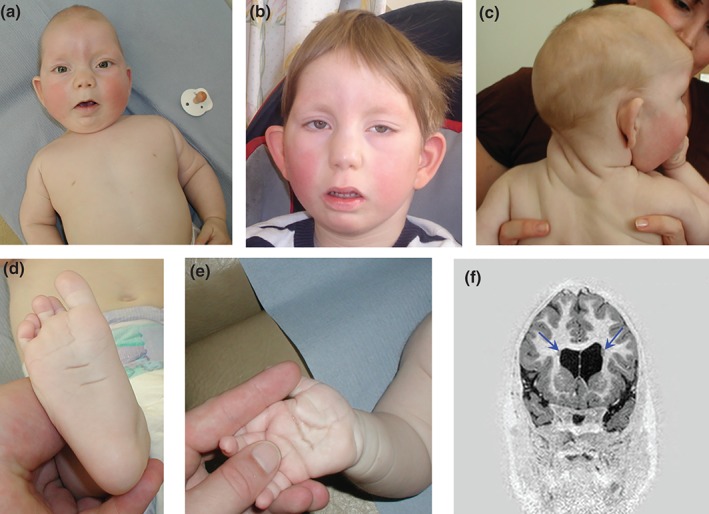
(a, b) Proband at ages 7 months and 8 years, respectively. Note the prominent metopic ridge, turricephaly, sparseness of lateral eyebrows with medial flare, long palpebral fissures, eversion of lower eyelids, low‐set prominent ears. (c) Thick, wrinkled skin on the neck posteriorly. (d, e) Deep plantar and palmar creases with fetal finger pads. (f) Coronal MRI image from inversion recovery volume sequence demonstrating two grey matter rounded nodules (blue arrows) adjacent to the borders of the lateral ventricles, in keeping with periventricular heterotopia. Written consent from the family to publish these images was obtained.
